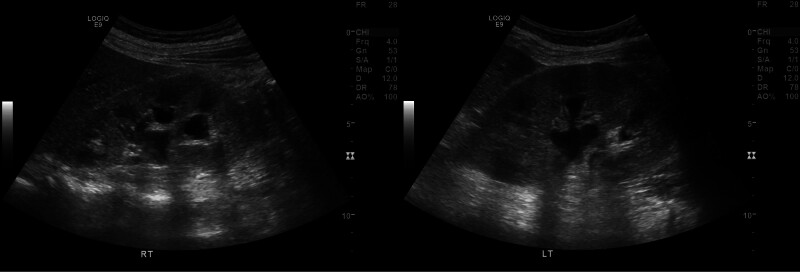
Abdominal ultrasound showing both kidneys of normal size (right: 11.26 cm, left: 11.36 cm) with slightly increased cortical echogenicity and bilateral hydronephrosis.
